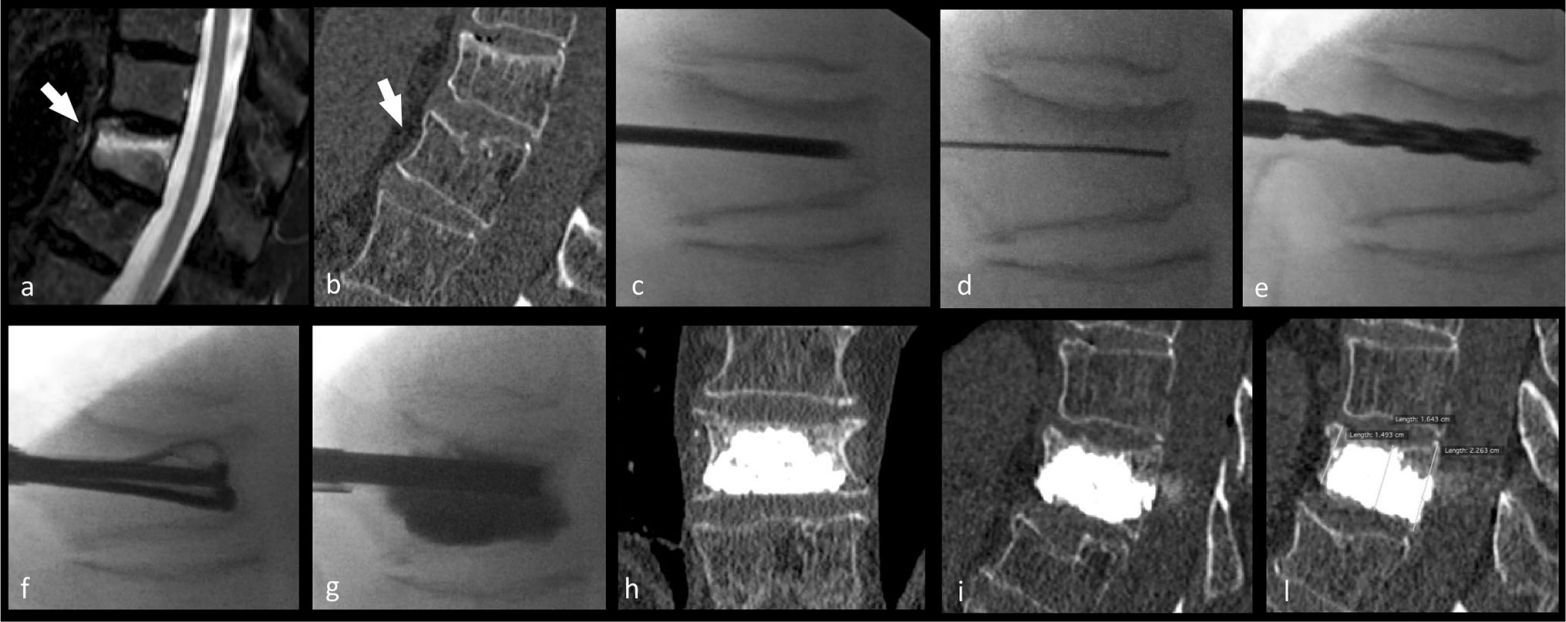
In this figure, the case of a 63-year-old female subject presented with acute back pain of 7 on the VAS. Panel a shows sagittal fat-saturation T2 weighted image and shows an A1.1 Magerl-type fracture at L2 (white arrow); Fig. 1b shows corresponding sagittal bone window CT image. In the panel c–g, the different phases of the Tektona bone remodeling procedure are given. In the panel c–g, the different phases of the Tektona bone remodeling procedure are given: c introduction of the trocar needle into the vertebral body; d the Kirschner wire has been introduced and the trocar needle removed; e the drill has been advanced over the Kirschner wire; f insertion of the VFR device and expansion of the lamella; g after the VFR device has been totally removed, the bone fillers were introduced and the bone cement has been injected. In the panel h and i, the postoperatively acquired CT is given, whereas in the panel l, the 12-month follow-up
